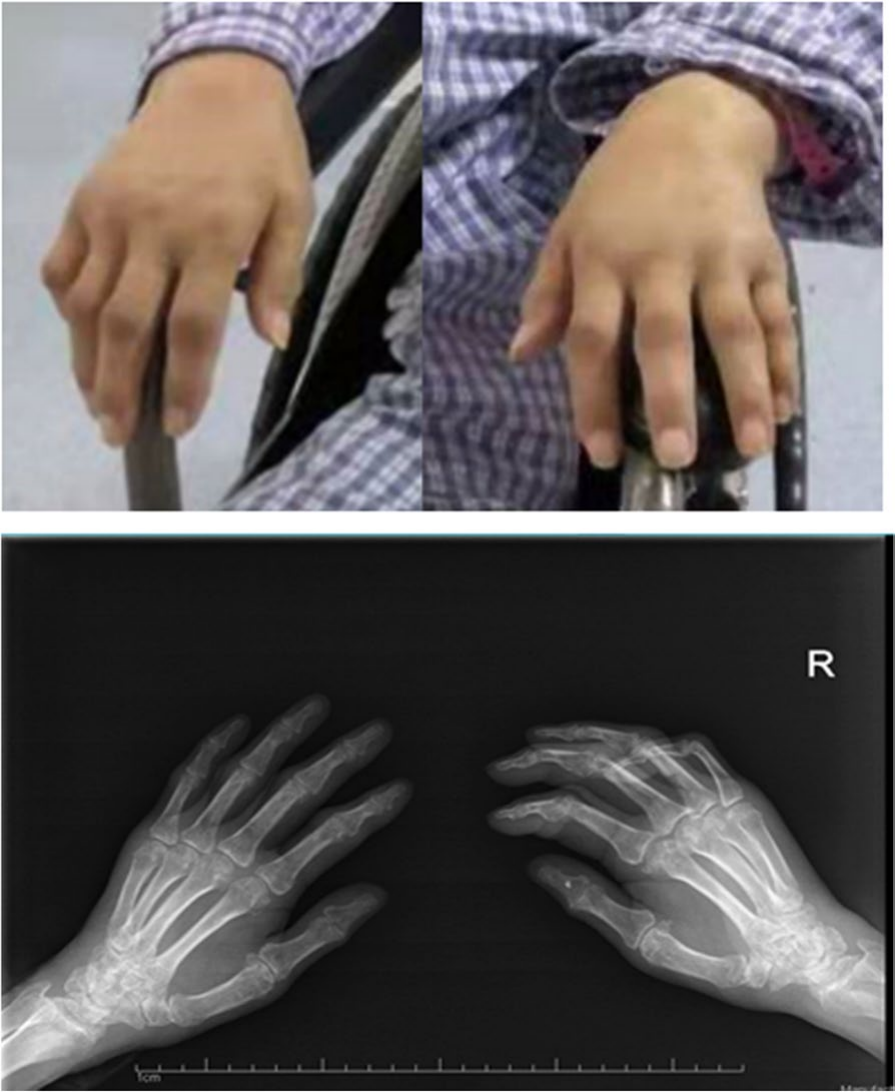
Charcot neuroarthropathy in hands after cervical central cord syndrome: six months after spinal cord injury, painless swellings (above) and degenerative changes of both proximal and distal interphalangeal joints at X-ray of the hands (below)
